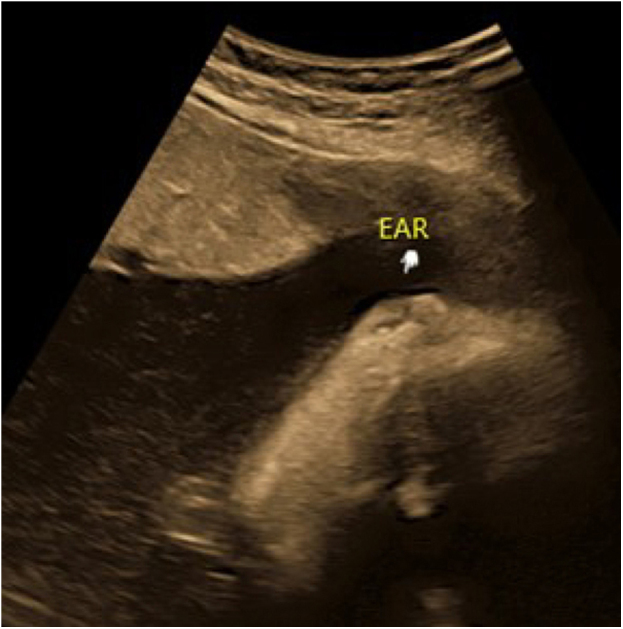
2-D ultrasound: microtia (arrow)
